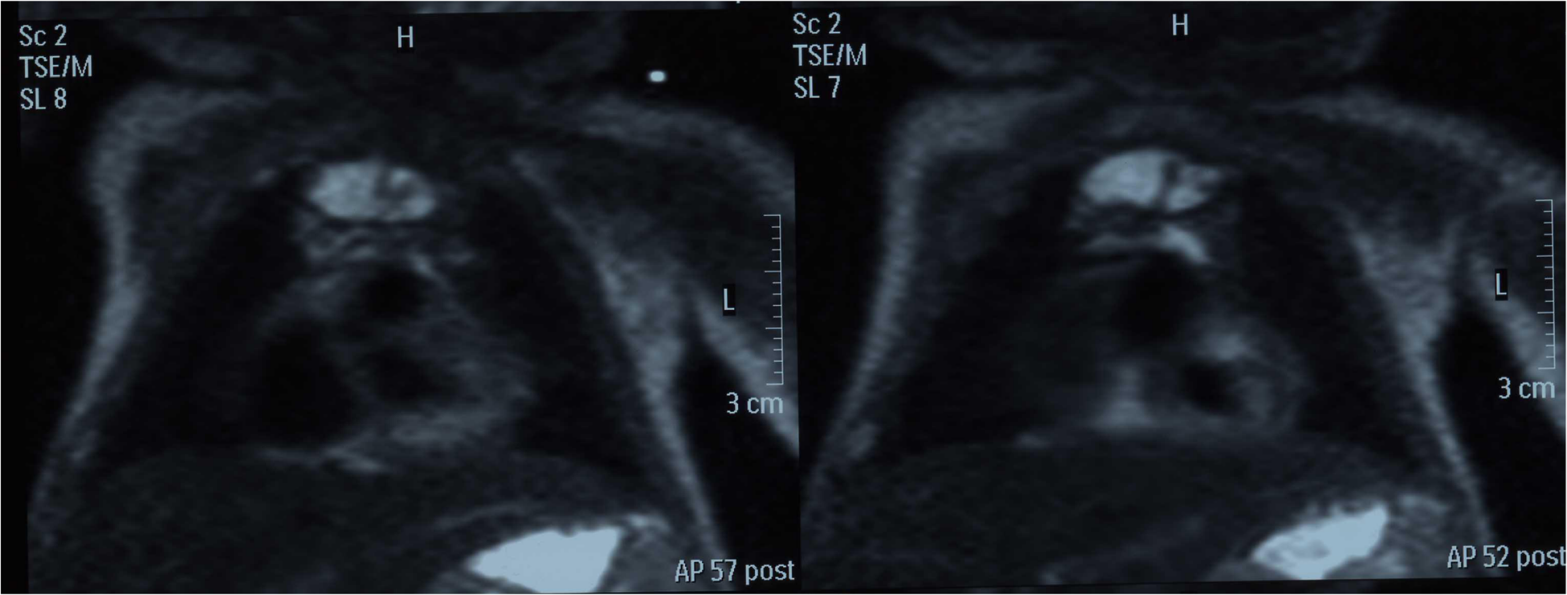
MR-sequences on day 16: thymus occupies the antero-superior mediastinum, with greatly reduced dimensions compared to the previous CT scan. Thymus appears to be homogeneous and with hyperintense signal in T1 as in T2 sequences. This aspect is suggestive of a recent bleeding. (Intera 1,5 T- Philps)
